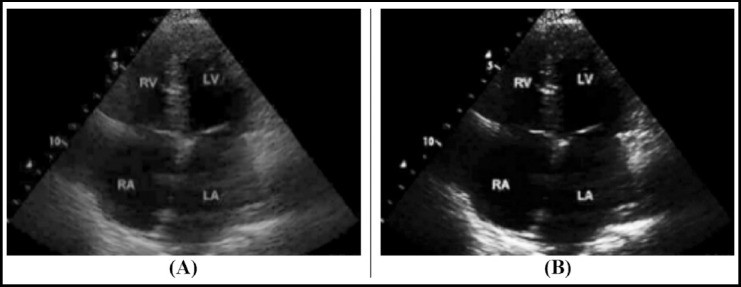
Comparison of echocardiography before and after optimization. Note: (A) was the image before optimization, and (B) was the image after optimization.
